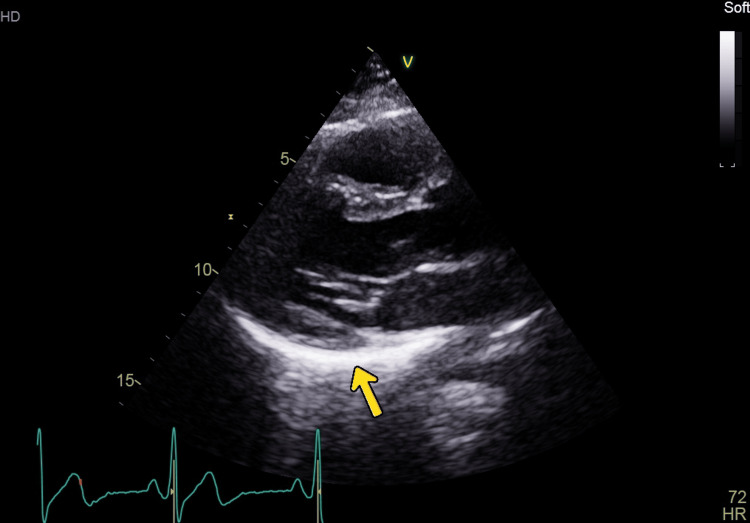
Echocardiogram suggestive of pericarditis
